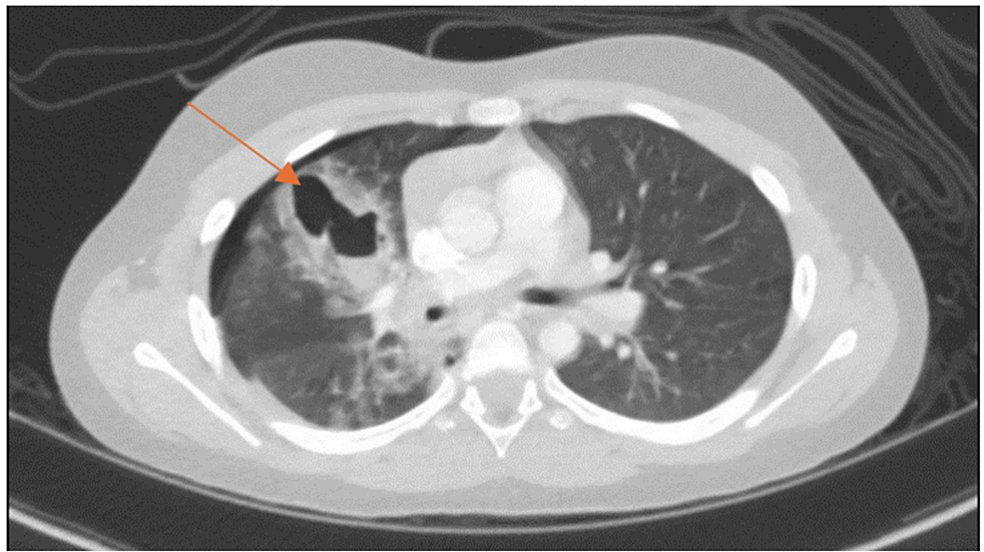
Transverse section CT of the thorax. Right-sided pneumothorax and patchy inhomogeneous airspace opacification in the right lung and multiple cavities with air-fluid levels. The arrow pointing to the cavity with the air-fluid level.
